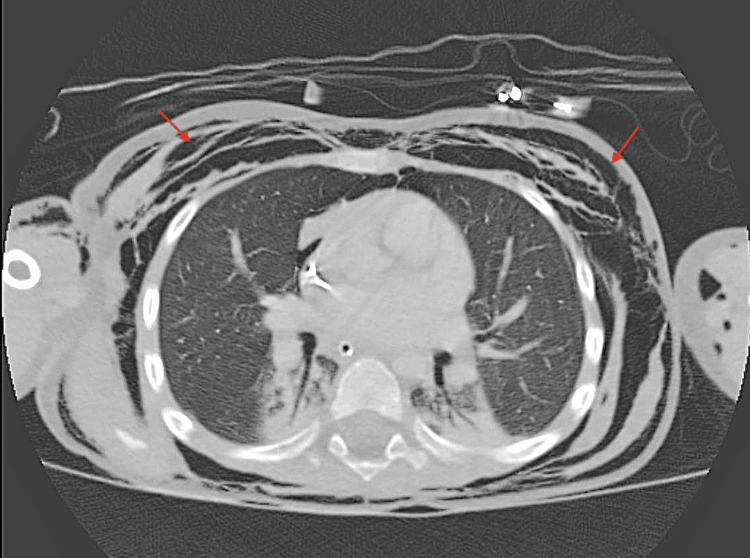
Axial CT Chest demonstrating extensive subcutaneous emphysema (red arrows) involving the anterior and lateral chest wall Air is seen dissecting through the soft tissues circumferentially around the thorax. This level demonstrates the most prominent extent of postoperative emphysema.
